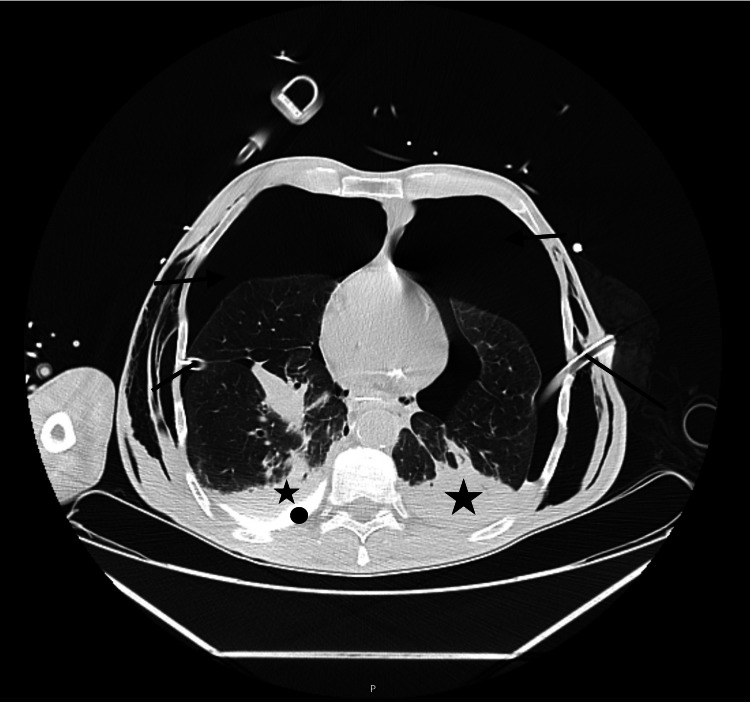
Second CT scan after the thoracic drainage technique Extensive high-volume bilateral hydrothorax (star) and pneumothorax (arrow) associated with pleural effusion (circle) are shown, as well as the bilateral thoracic drainage tubes in the pleural cavity (line).
